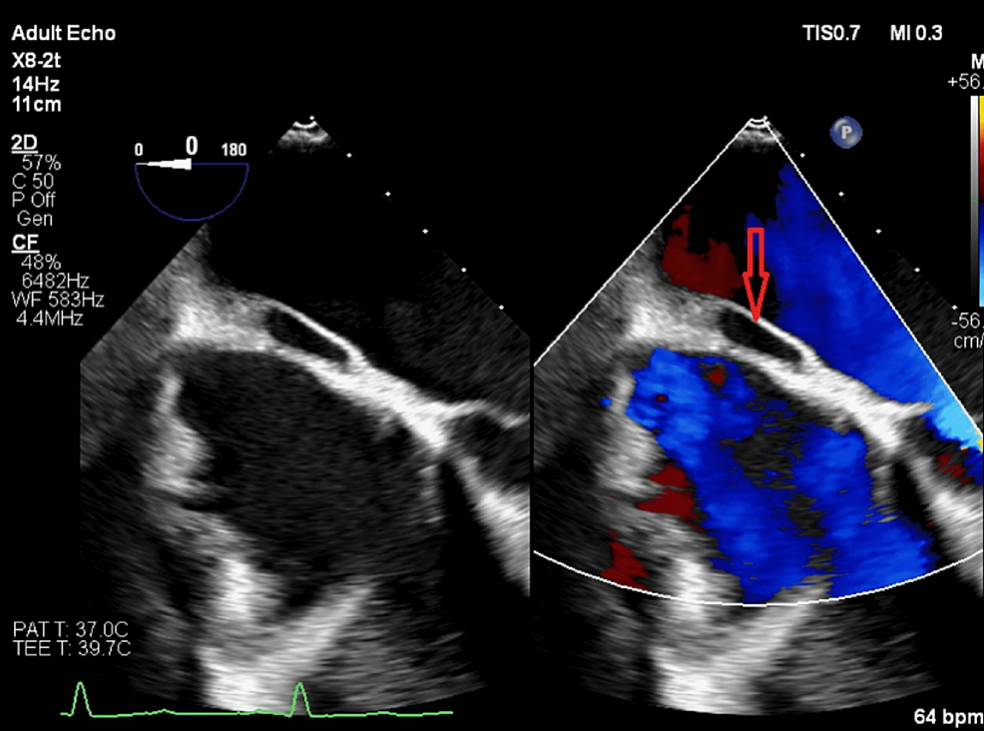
TEE four-chamber view at 0 degrees with a focus on the atrial septum (red arrow). The color Doppler flow shows no communication with the pouch TEE: transesophageal echocardiogram
